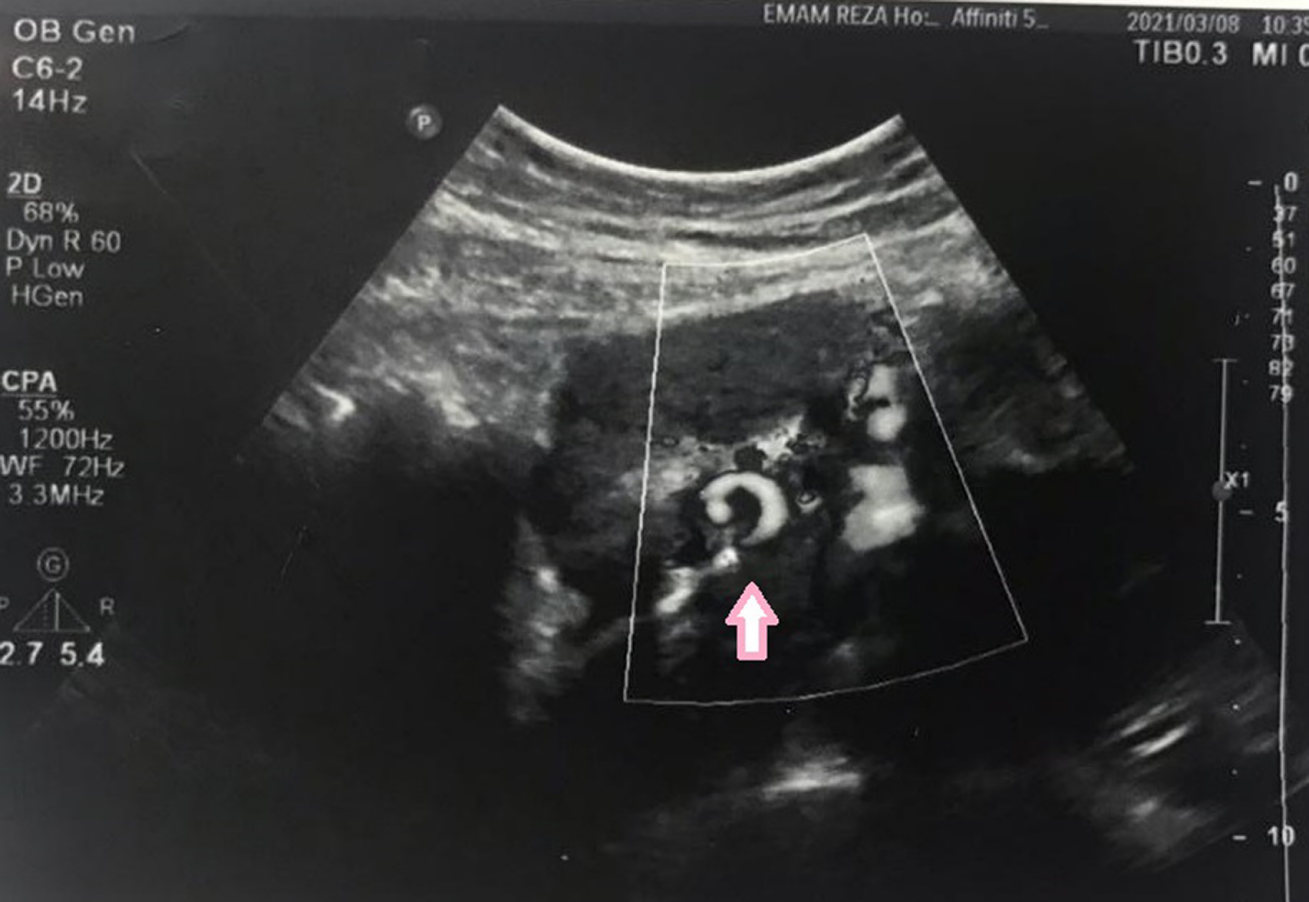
Whirlpool sign (pink arrow) which presented as twisted circular vessels on Doppler
